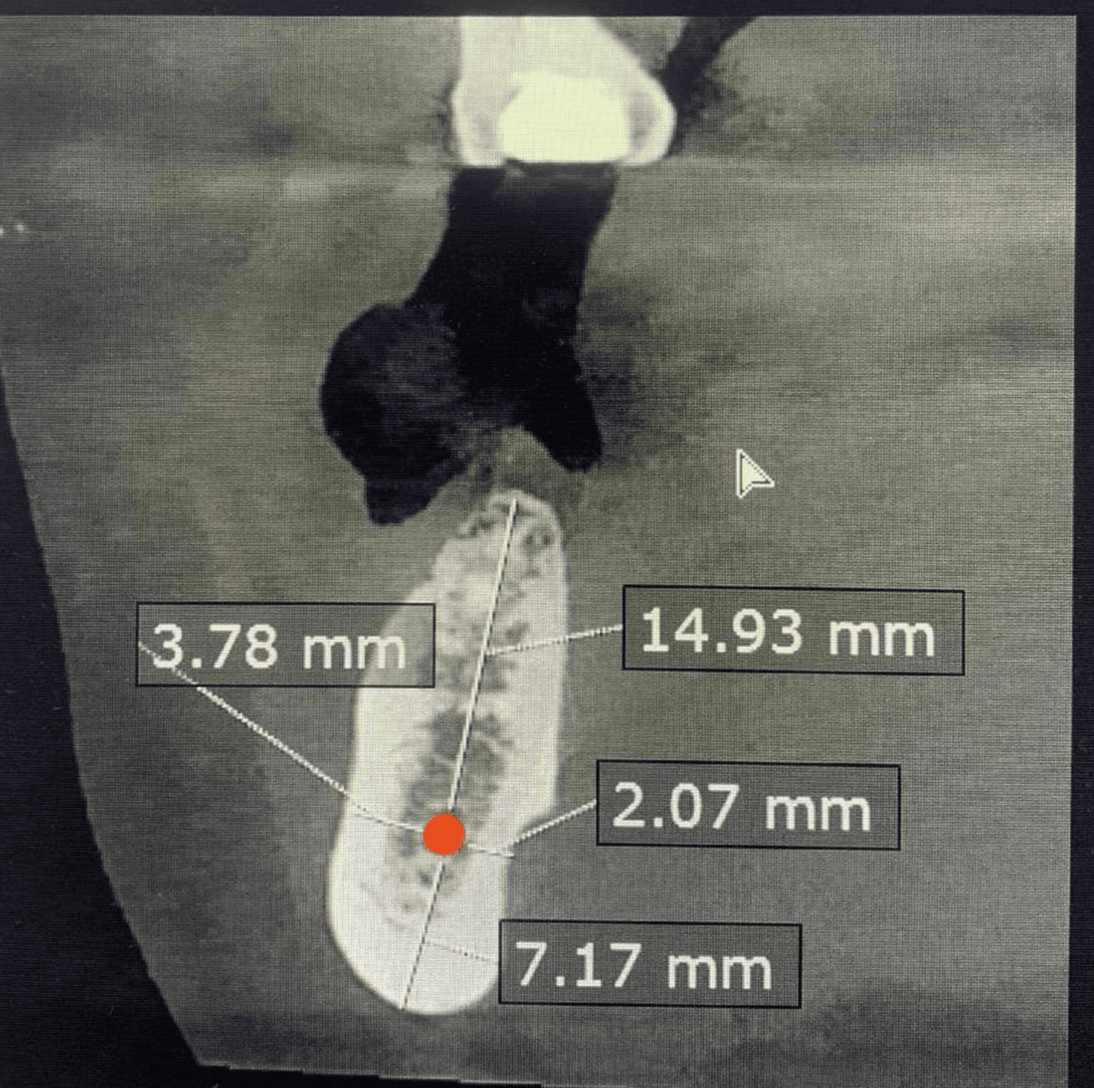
A sagittal cut from the original CBCT image showing the measurements of the body of the mandible by Ruler tool surrounding the IAC. Body vertico occlusal (14.93 mm), body vertico apical (7.17 mm), body horizontal buccal (3.78 mm), and body horizontal lingual (2.07 mm). CBCT: cone-beam computed tomography; IAC: inferior alveolar canal
